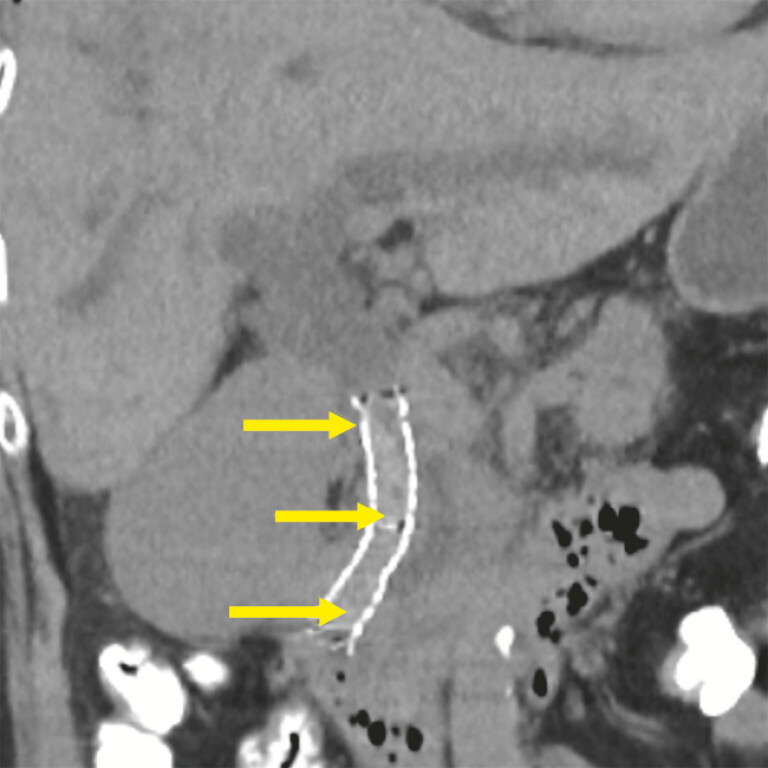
A computed tomography image taken 2 days after the index procedure. The yellow solid arrows indicate a high-density embolus suspected to be blood clot impaction along the entire length of the fully expanded MH-C-SEMS. MH-C-SEMS, multi-hole covered self-expandable metal stent.
